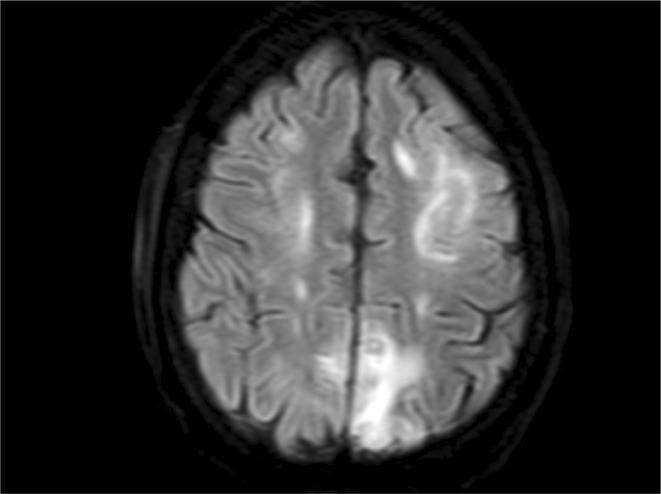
FIGURE 1 Bilateral parasagital hypersignal noted, FLAIR.
